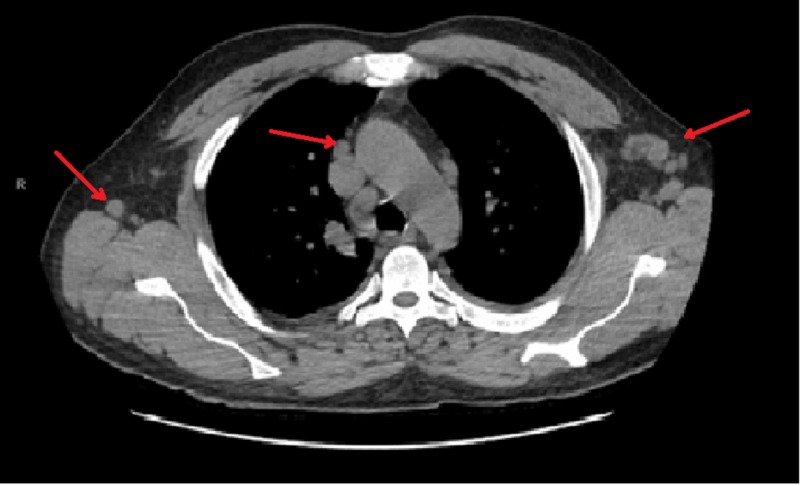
CT scan of the chest showing enlarged mediastinal and axillary lymph nodes.
